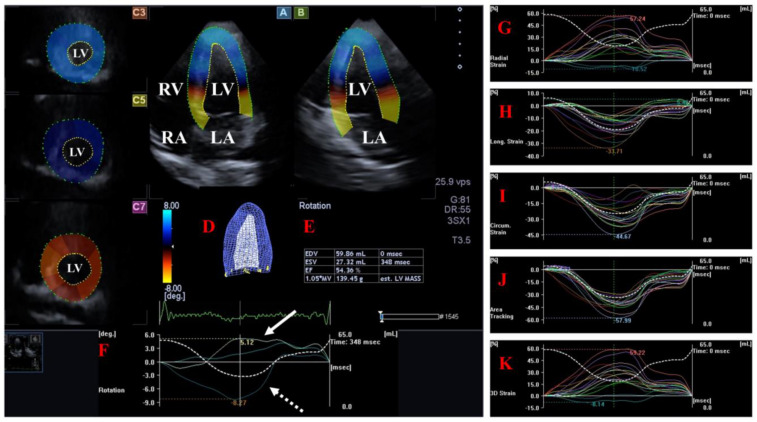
Examination of the left ventricle (LV) by three-dimensional (3D) speckle-tracking echocardiography. Following echocardiographic data acquisitions, the following typical views are created: (A) Apical 4-chamber and (B) two-chamber longitudinal views and (C3,C5,C7) cross-sectional views at apical, midventricular and basal levels, respectively. LV can be easily detected alongside other heart cavities including the left atrium (LA) and the right atrium (RA) and ventricle (RV). A number of other details were also presented including (D) 3D cast and (E) end-diastolic (EDV) and end-systolic (ESV) volumes of the LV together with ejection fraction (EF) and mass of the LV and (F) curves representing changes in volumes and strains of LV over time. (F) Apical [white arrow] and basal [white dashed arrow] LV rotations and (G) radial, (H) longitudinal, (I) circumferential, (J) area and (K) 3D strains are also demonstrated.
